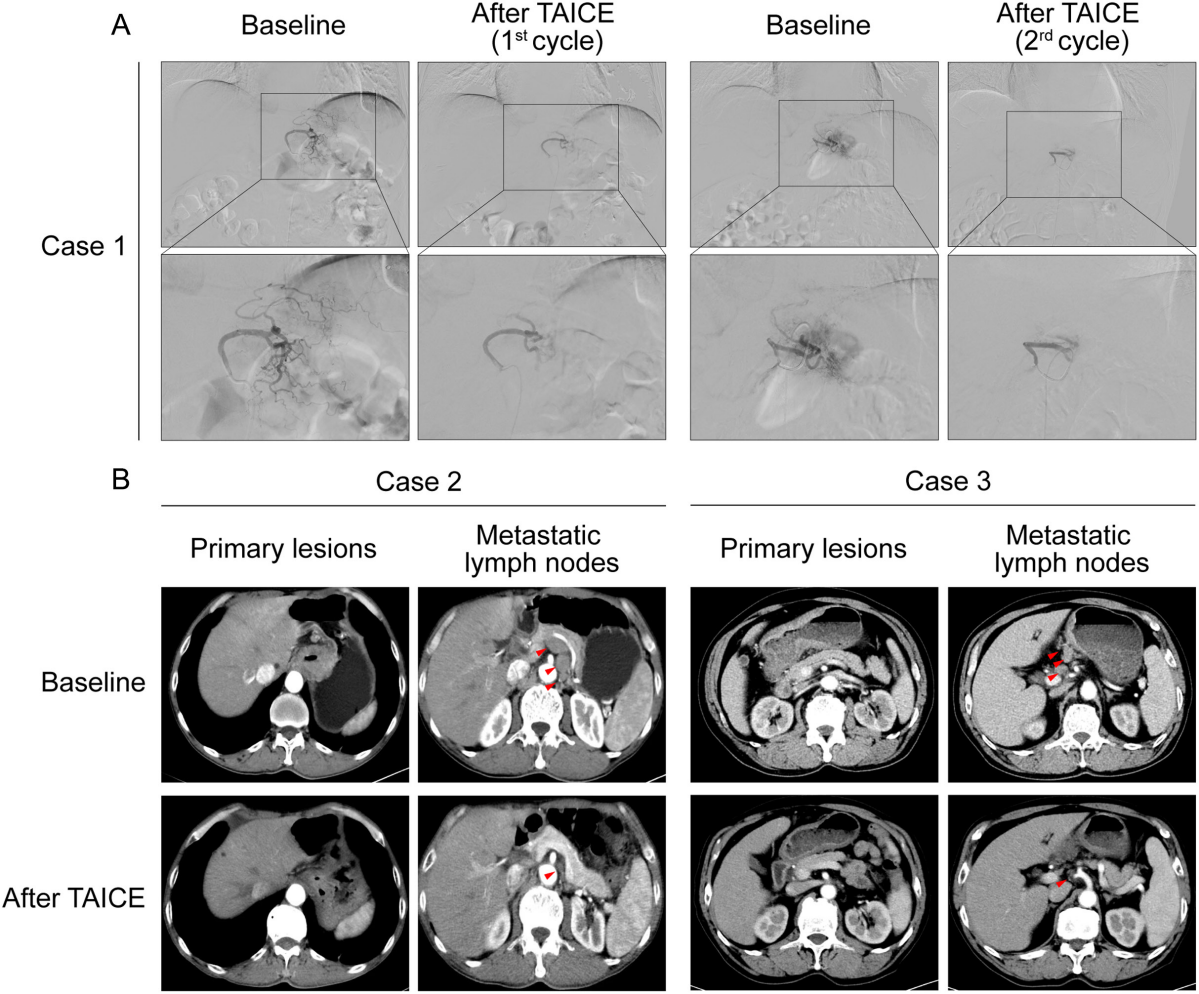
(A) Representative digital subtraction angiography (DSA) images of tumor feeding artery of gastric cancer from one patient at baseline and after TAICE in two cycles of TAICE. (B) Representative computed tomography (CT) images of primary lesions and lymph node metastases of gastric cancer from two patients at baseline and after TAICE. Red arrows indicated lymph node metastases.
